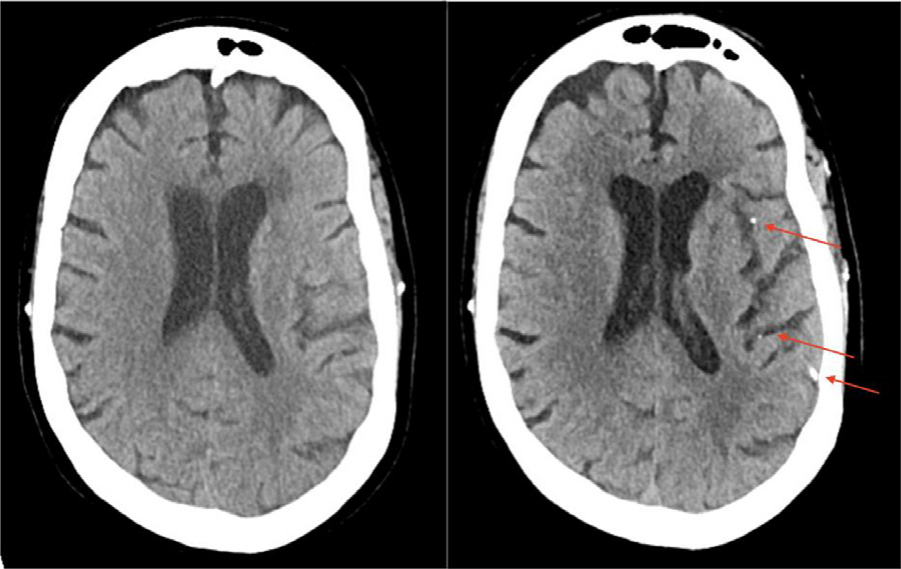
Initial unremarkable axial CT head without contrast on presentation (left); axial MIP reconstruction of follow-up CT head without contrast performed 13 h later showing numerous, new calcified emboli on a single MIP image, with additional calcifications seen on other slices (right).
